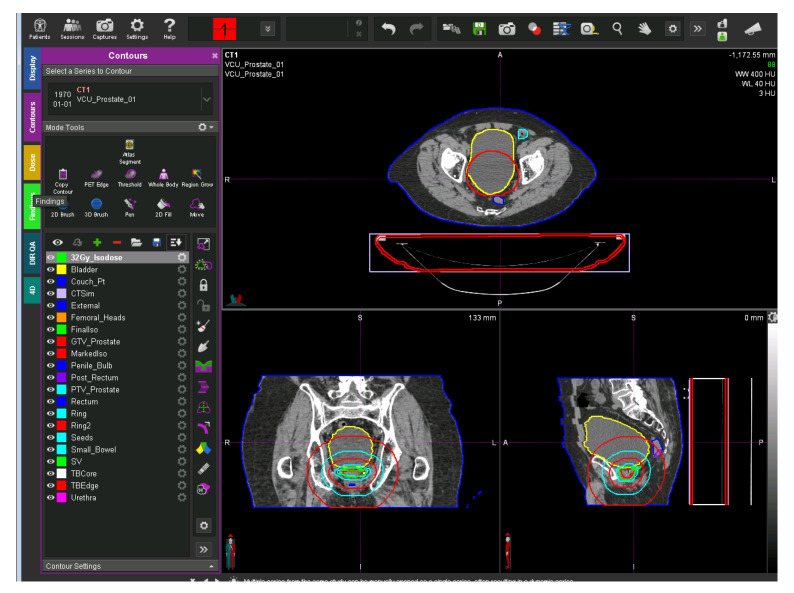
A representative CT image overlaid with its defined structures. The left side of the figure shows the physician-transcribed names of the structures delineated on the right side. The physician-transcribed names and structures delineated can be matched by the color.
